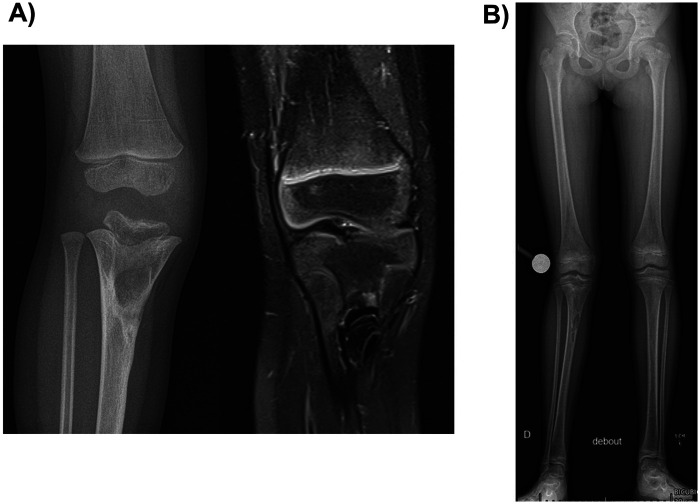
(A) follow-up radiograph and MRI demonstrating progressive restoration of the proximal tibial epiphysis toward its normal position, with normalisation of its shape and resolution of the cupping deformity. (B) Standing long-leg radiograph obtained 10 years after surgery showing tibial overgrowth associated with a mild valgus deviation of the right lower limb.
